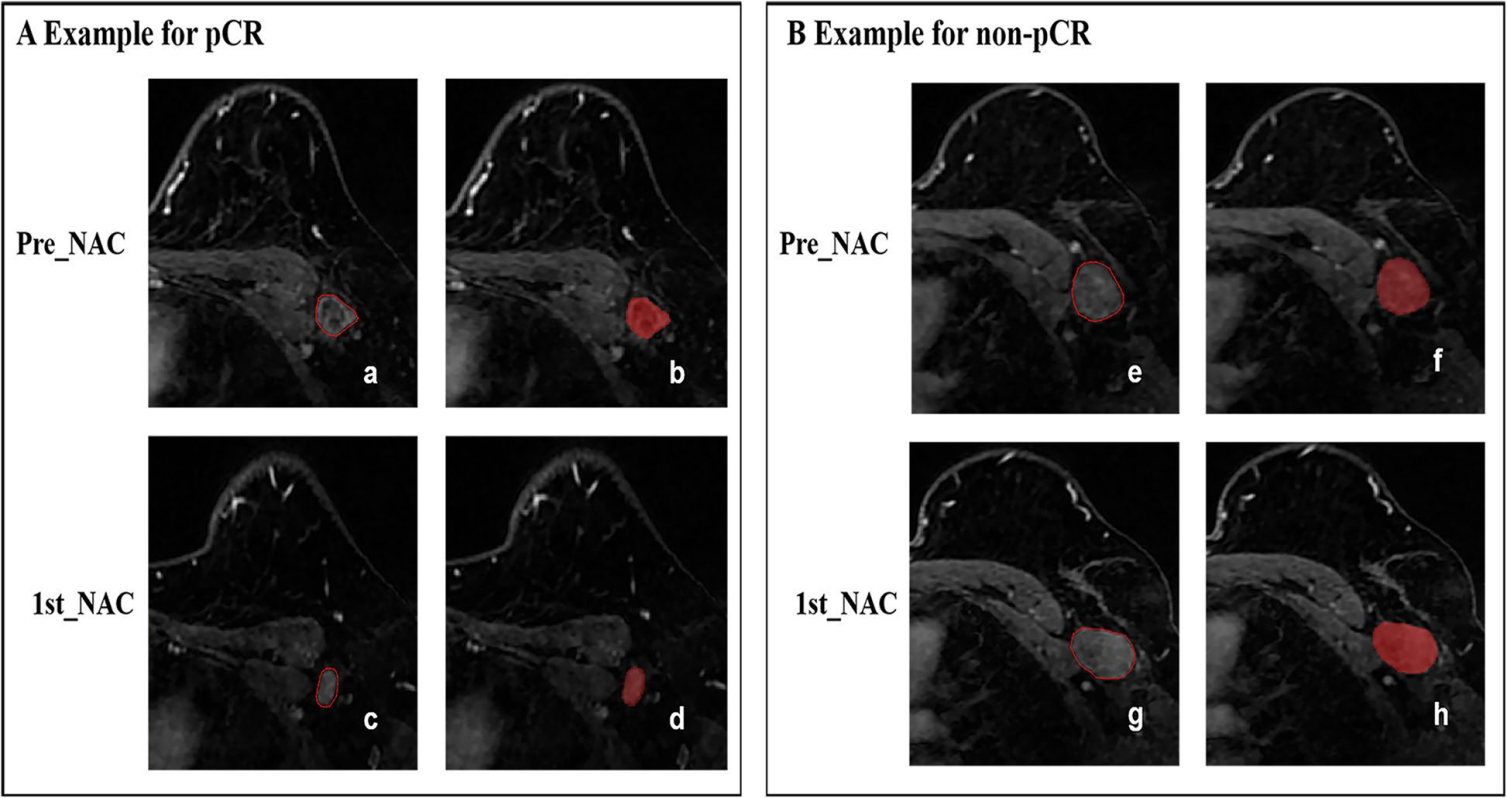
Representative images segmentation. A Images from an axillary pCR breast cancer patient (aged 34 years old with invasive ductal carcinoma of metastasis in the left ALNs). B Images from an axillary non-pCR breast cancer patient (aged 55 years old with invasive ductal carcinoma of metastasis in the left ALNs). a-b and e-f were the baseline DCE-MRI; c-d and g-h were the first cycle DCE- MRI). ALN axillary Lymph node, DCE dynamic contrast enhanced, apCR axillary pathologic complete response
